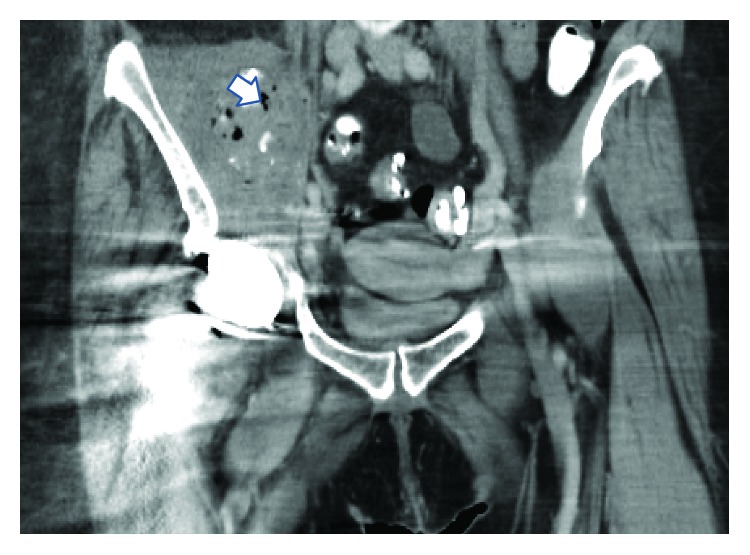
CT: right iliopsoas abscess with calcifications and air (arrowhead).
